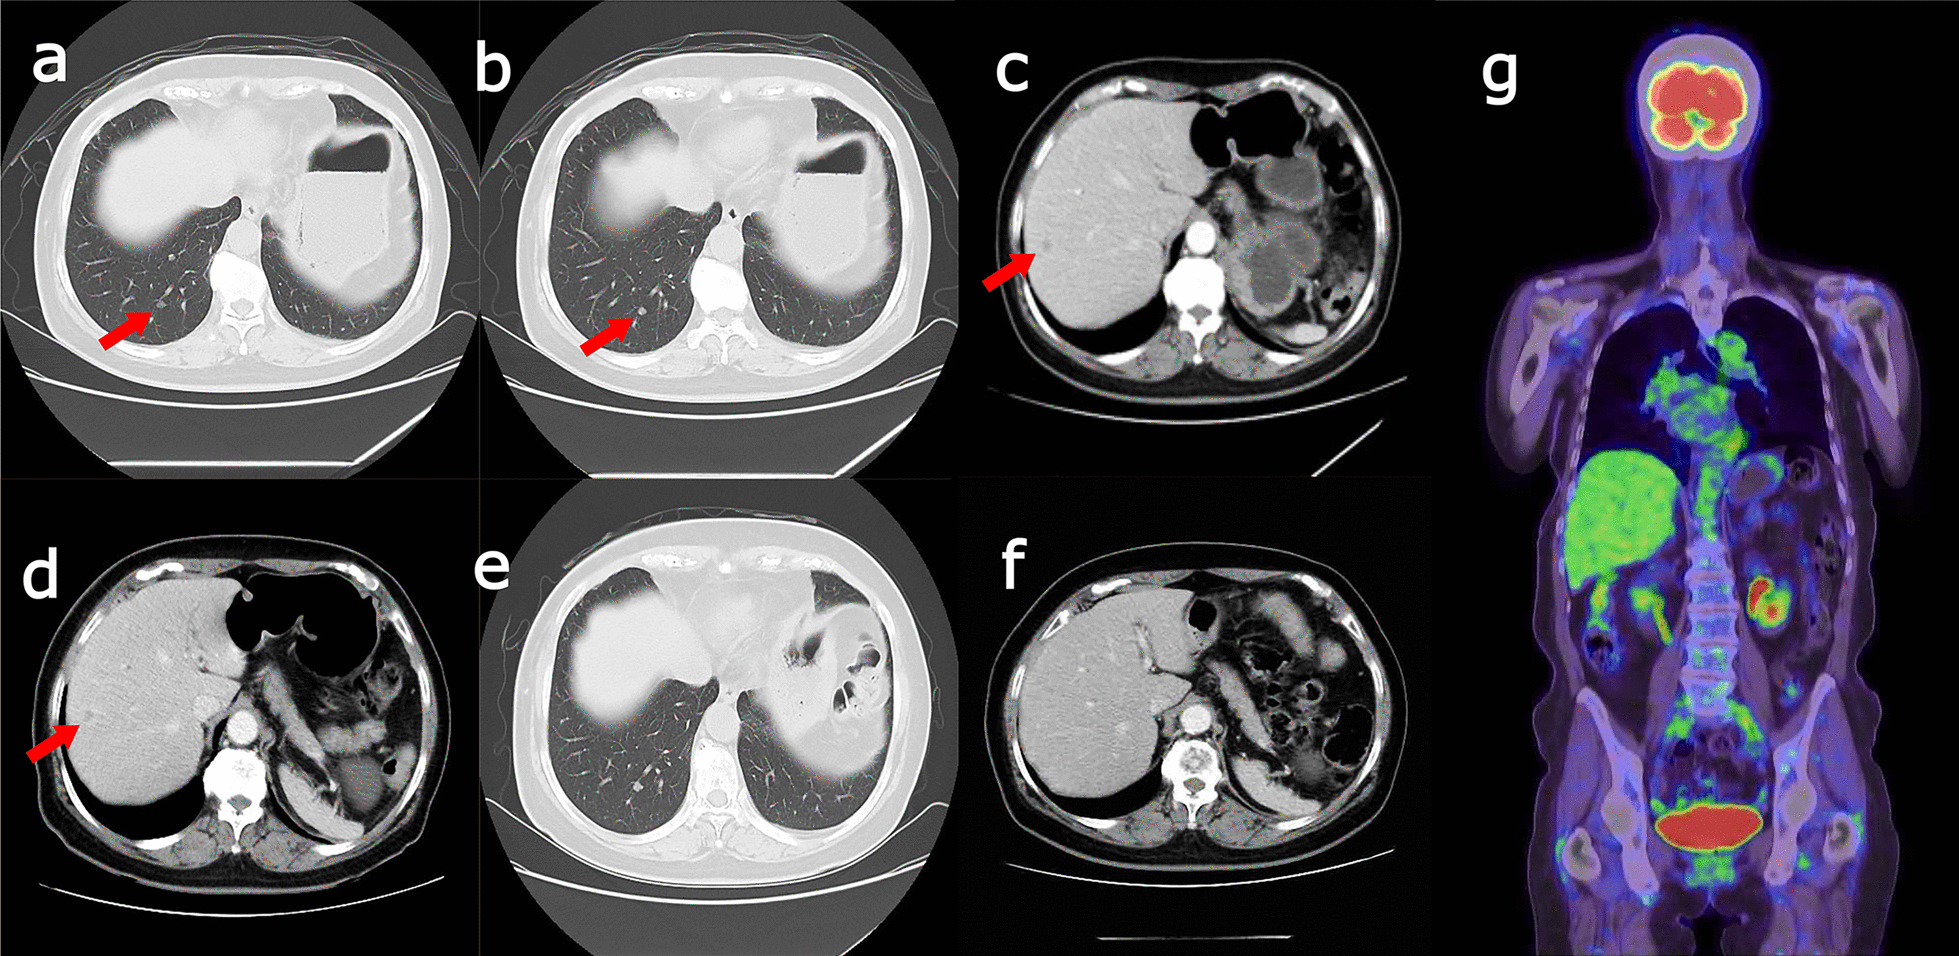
Images at the time of the patient’s second recurrence. Computed tomography showed two metastases to the inferior lobe of the right lung (a: arrows) and a small metastatic lesion in the liver (b: arrows). The liver metastasis had slightly increased 7 months after the second recurrence (c: arrows). At 4 months after the start of palbociclib, the liver metastases had receded slightly (d: arrows). At 7 months after the start of palbociclib, the lung metastasis had disappeared completely (e). At 1 year and 5 months after the start of abemaciclib, the liver metastasis had completely disappeared (f). Integrated positron emission tomography–computed tomography showed no metastasis or recurrence in any organ, including the lungs and liver (g)
